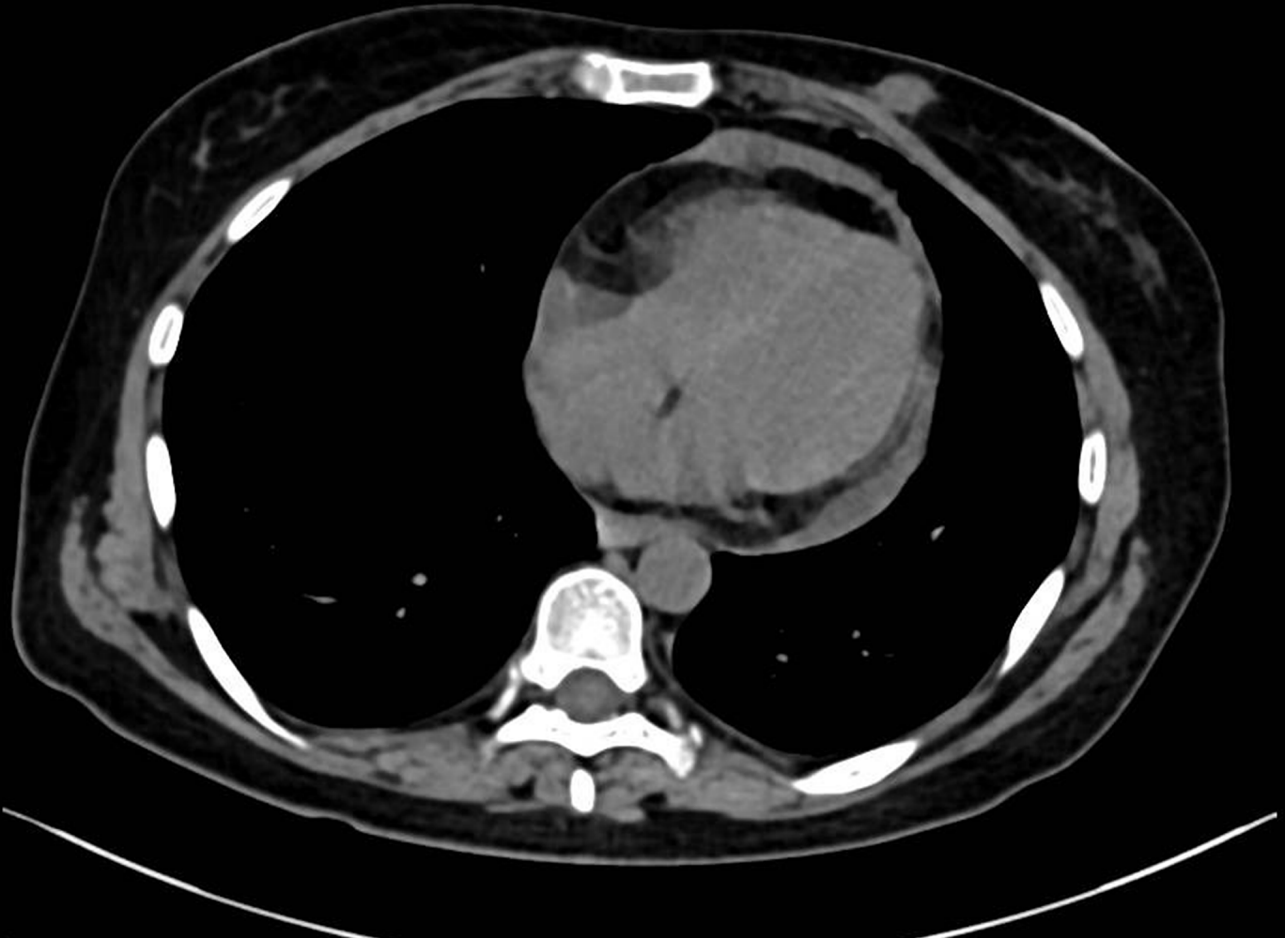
Chest CT after treatment.
